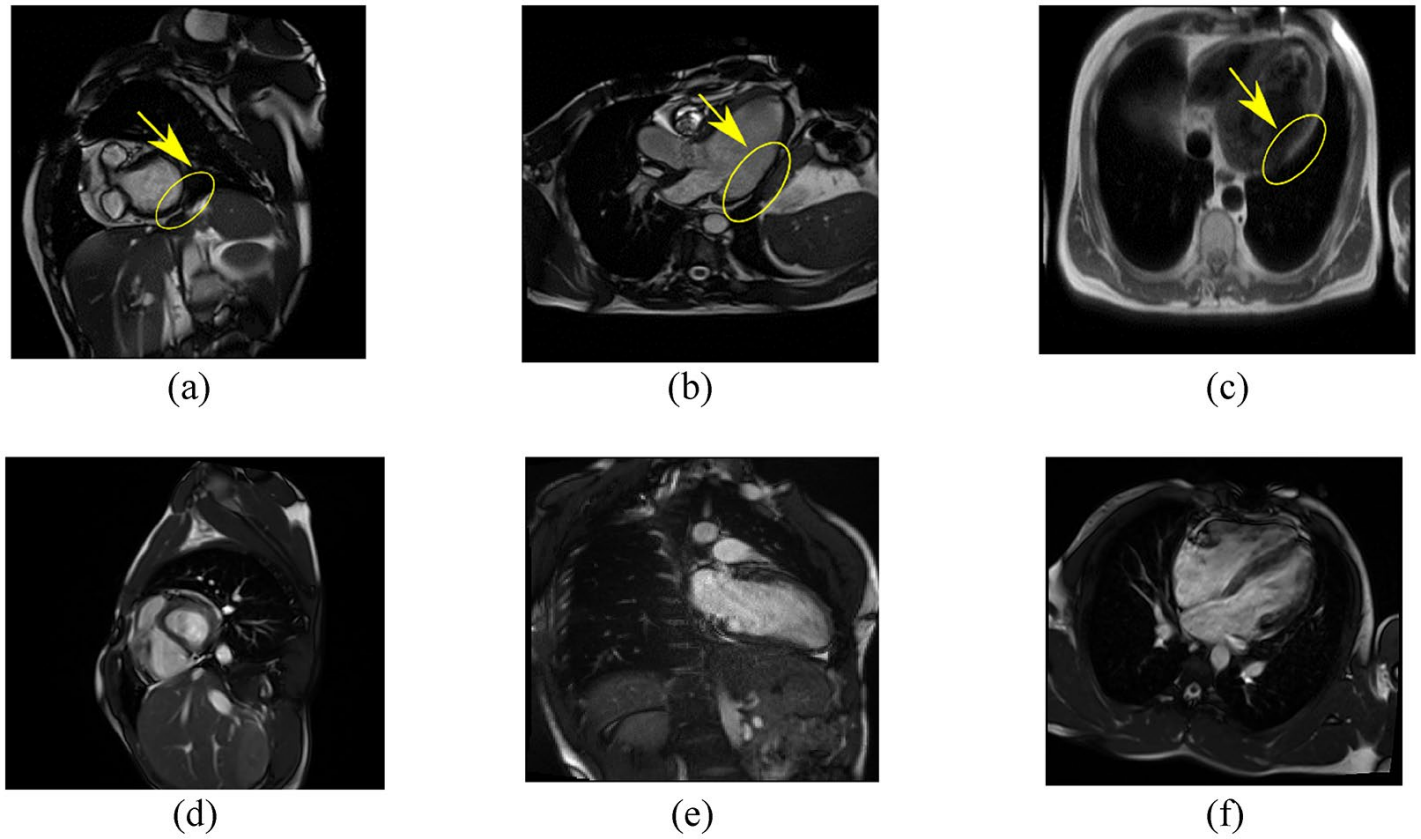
Example cardiac magnetic resonance images from coronary artery disease patients (a–c) and healthy subjects (d–f). c is a black-blood spinecho image; the rest are single-phase images of steady-state free precession CINE images. The lesions indicating CAD have been marked with yellow color in parts (a–c).
